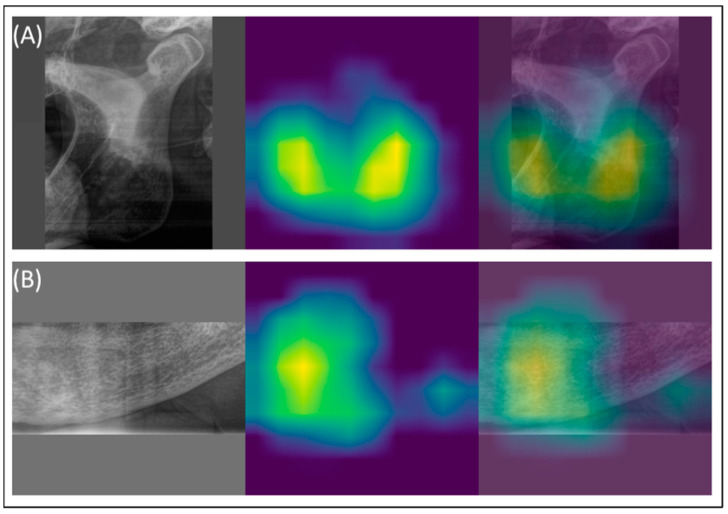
Examples of true positive predictions are shown in (A) for osteoporosis detection in a panoramic radiograph with the cropped angulus and (B) for osteoporosis detection in a panoramic radiograph with the cropped mental foramen.
